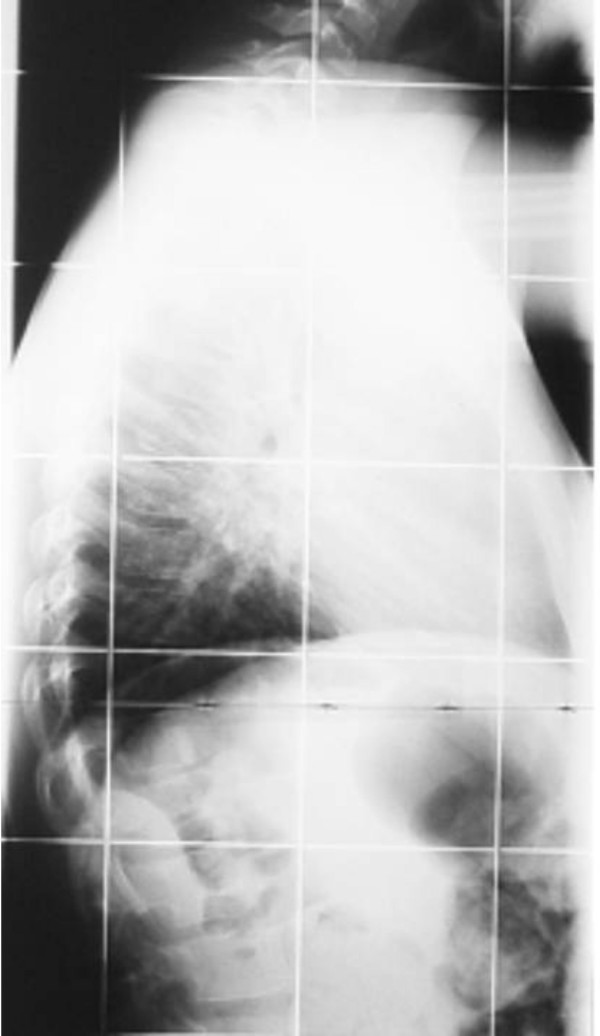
Lateral spine radiograph showed significant thoracic kyphosis secondary to malsegmentation of the upper thoracic vertebrae associated with scalloping of the posterior-end plates of the lumbar vertebrae. There was exaggerating lumbar lordosis associated with thoracic kyphosis.
